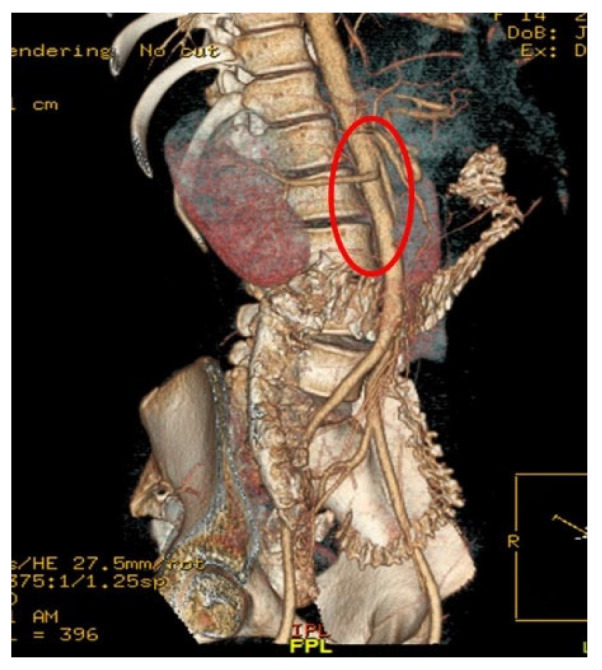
CTA: The aortomesenteric distance measured 4 mm.
